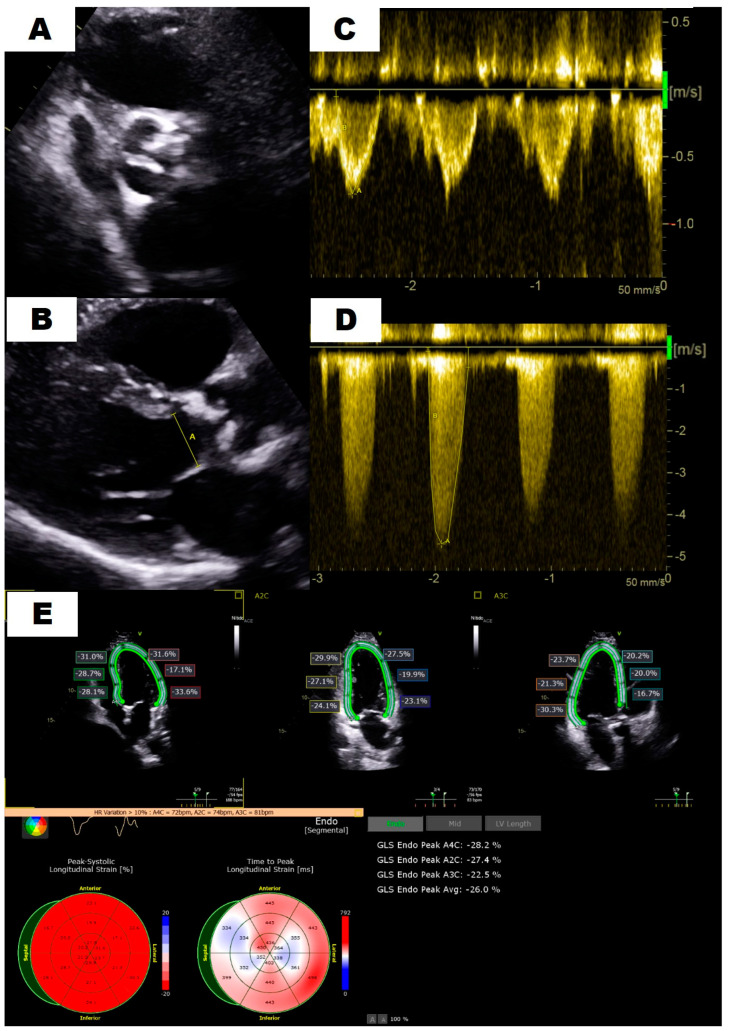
Comprehensive evaluation of aortic stenosis severity. An initial morphologic evaluation of the aortic valve in a short axis view (A) is crucial for careful measurement of left ventricular outflow tract diameter (B). Pulsed (C) and continuous (D) Doppler allow for the estimation of flow and aortic valve gradient, respectively. Finally, a comprehensive evaluation may include left ventricular strain analysis (E).
